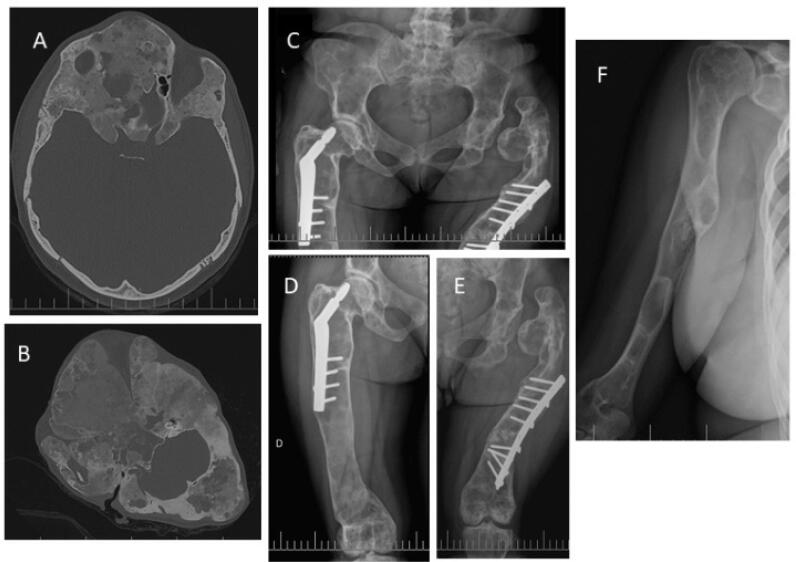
Images of patients with FD showing typical findings. ( A-B ) Axial computed tomography images of two different patients with craniofacial involvement, with typical “ground glass” appearance of affected bones and areas of heterogeneity and radiolucency. Severe enlargement of the frontal and occipital regions and thickened cranial base secondary to FD is evident in panel B. ( C-E ) Radiographs demonstrate extensive involvement of the pelvis and lower extremities, with severe Shepherd's crook deformity of both femurs, cortical thinning, sclerotic lesions, and “ground-glass” radiolucency. Plate and nail implants were placed in bilateral femurs. ( F ) Right humerus radiograph of a woman with monostotic FD showing sclerotic-appearing FD lesions. FD: fibrous dysplasia.
